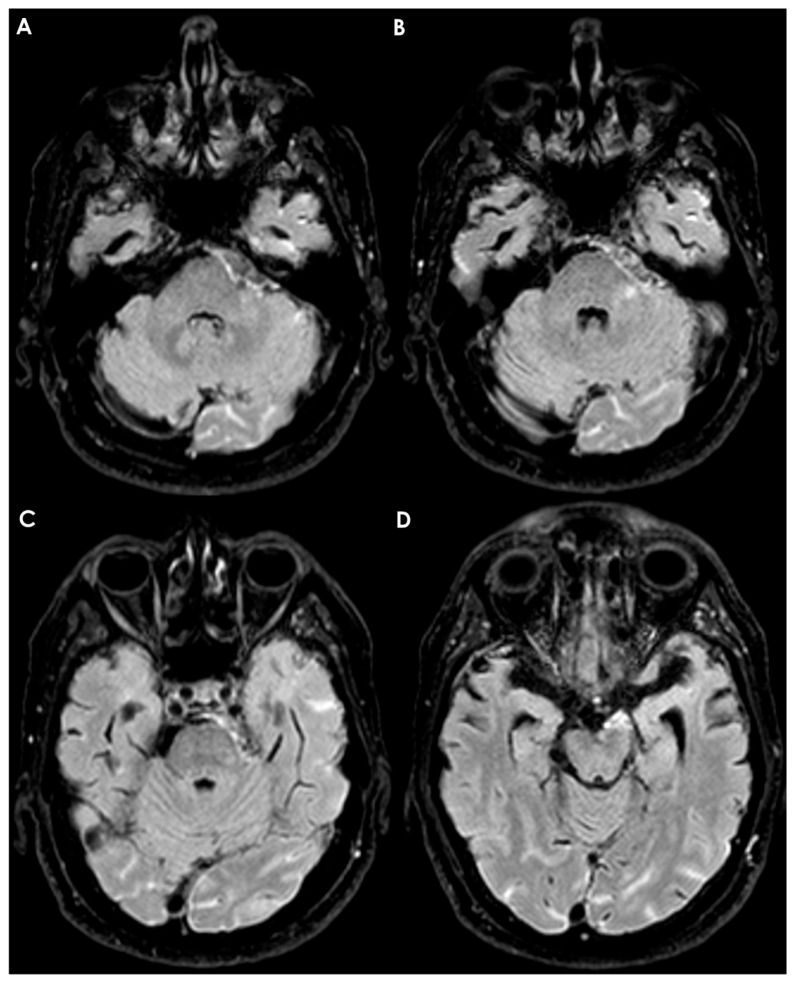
Axial 2D Fluid Attenuated Inversion Recovery (FLAIR) MRI (1.5 T scanner) in ascending slices from the pons to the midbrain (from (A–D)) showing a compartimentalized irregularly hyperintense signal in the left cerebellopontine cistern, corresponding with subarachnoid bleeding, associated with diffuse sulcal hyperintensities coming from the same source. The patient was a 64-year-old man with a spontaneous subarachnoid bleeding and a negative catheter angiography.
